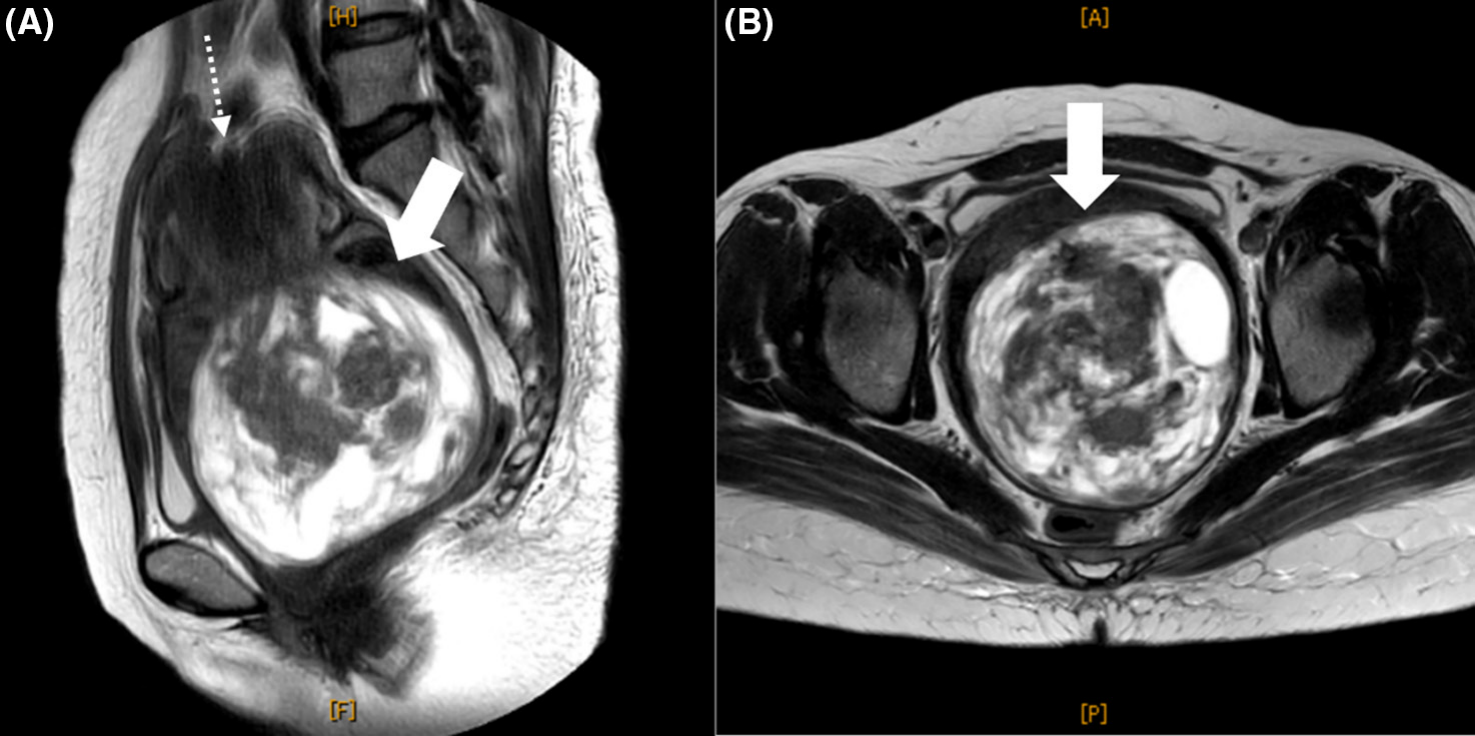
Magnetic resonance imaging showing uterine inversion caused by fibroid delivery. (A) A 10‐cm‐sized nodule in the vagina and “V shape” uterine fundus. (B) Layers of the inverted uterus with “bull's‐eye” configuration.
